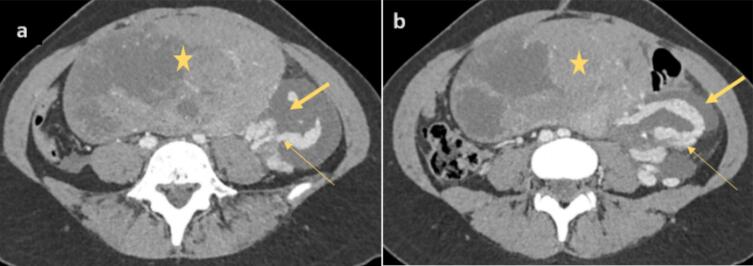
(a) and (b): CT slices at the abdominal level showing a mass (star) effect on the left ovarian pedicle with tortuous dilation of the ipsilateral ovarian vein (large arrow) and a lymphocele (small arrow).
